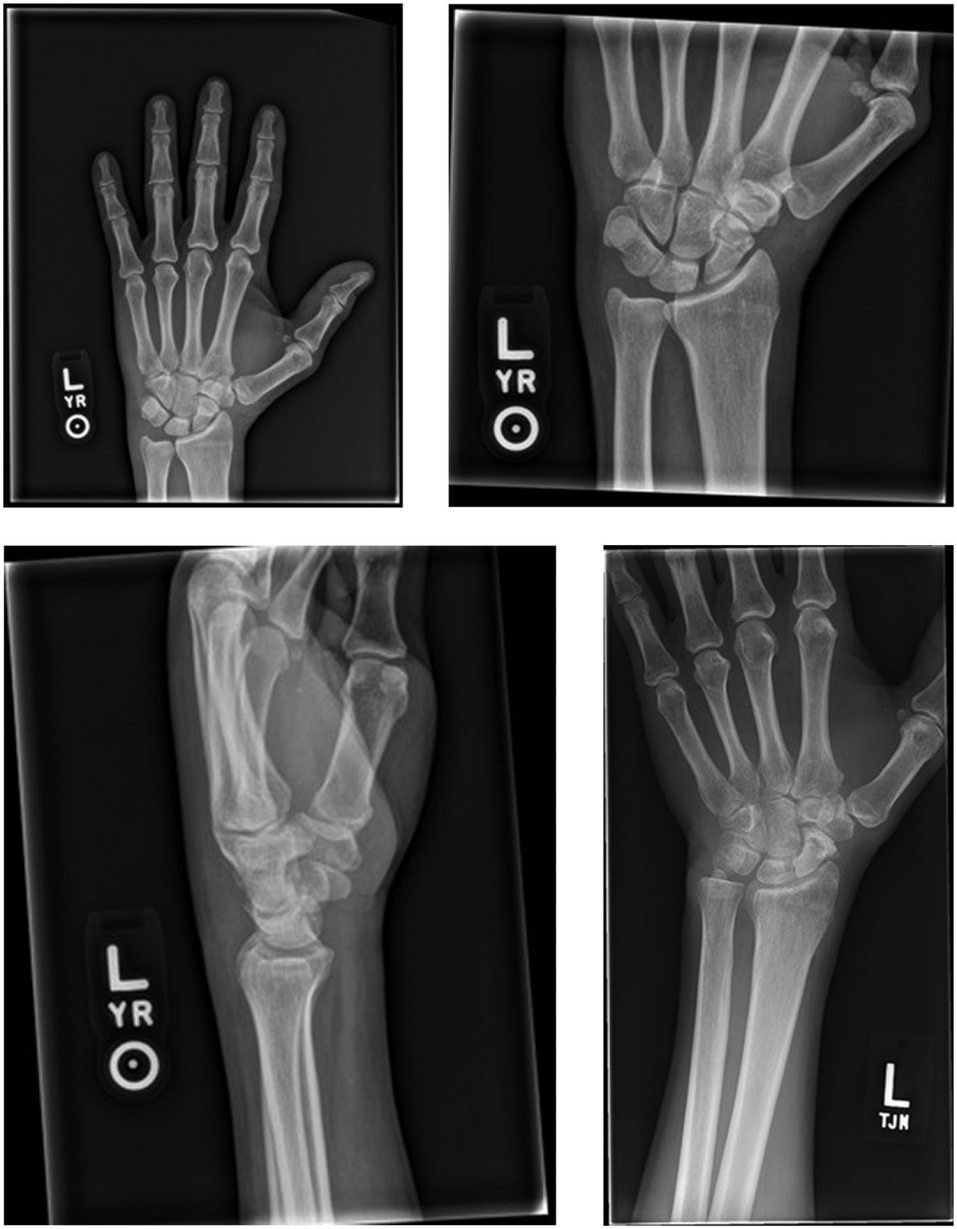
X-rays of the left hand and wrist at 2.5 year follow-up showing resolution of the subcutaneous air with no obvious sequelae. A new unrelated scaphoid fracture is noted.
